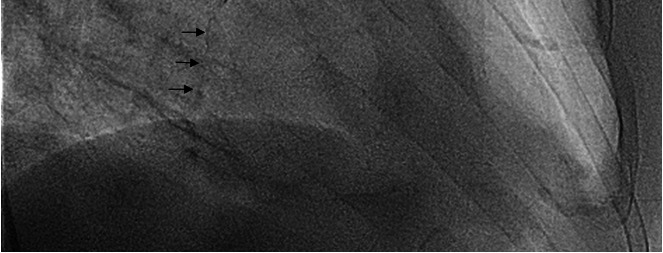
Right coronary artery – vascular calcification on plain radiography
